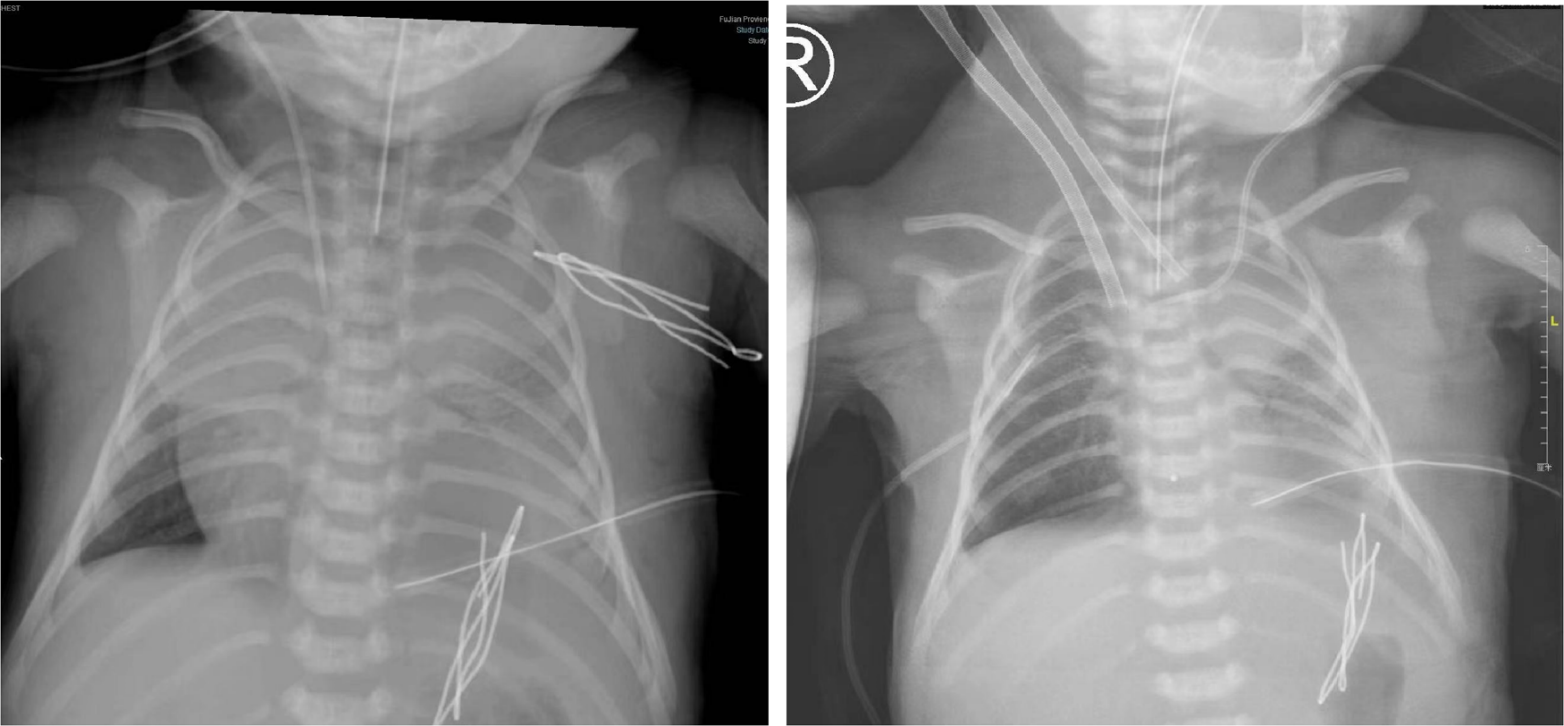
x-ray before ECMO treatment and during ECMO treatment in case 2.
